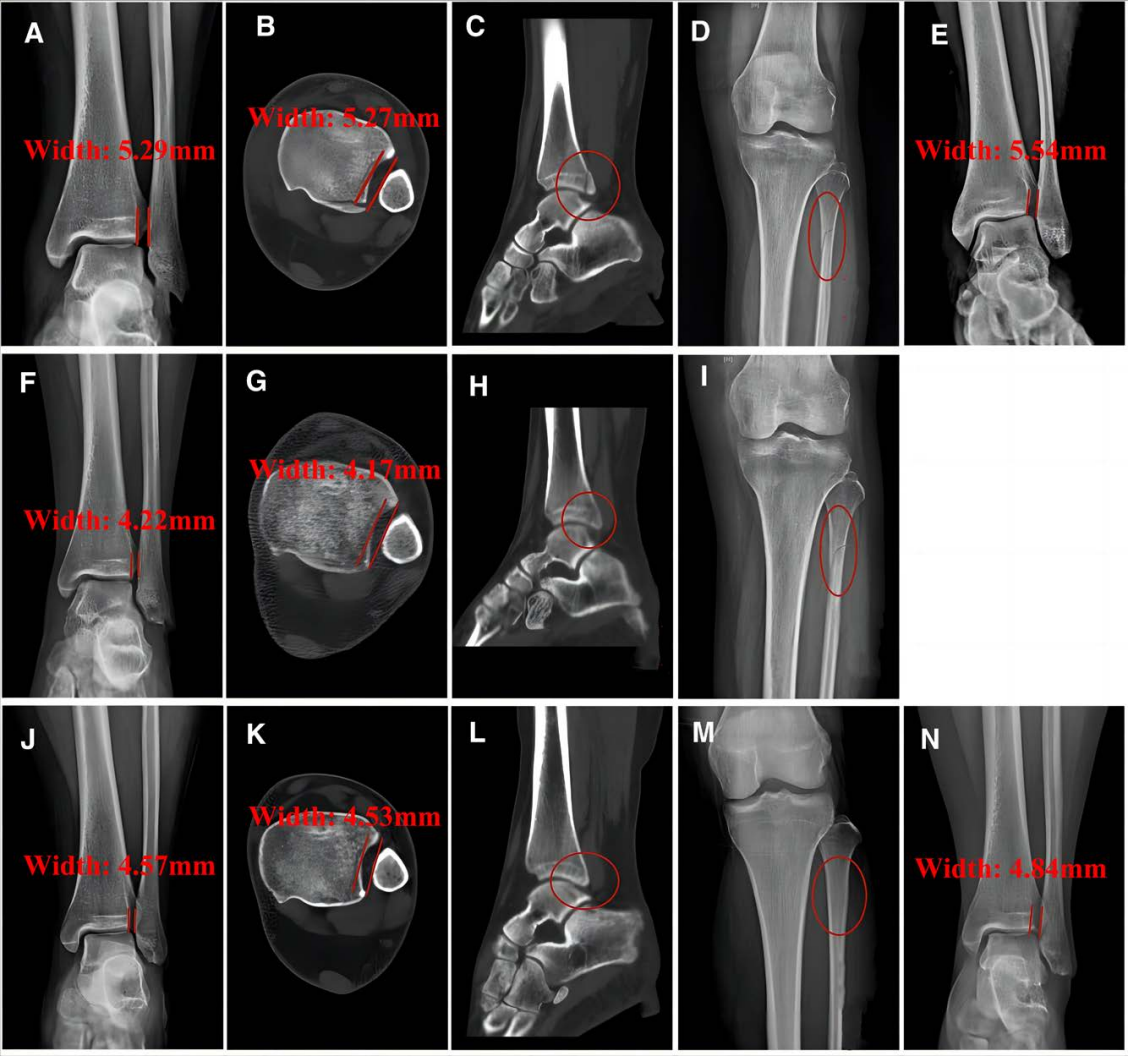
Typical imaging of the patient. (A–E) Radiography and CT scans at the first visit: (A) and (E) represented the non-stress and stress views, respectively; the width of the inferior tibiofibular space was 5.29 mm and 5.54 mm, respectively; (B) showed 5.27 mm in width of inferior tibiofibular space; (C) showed a fracture of the Volkmann tuberosity; (D) showed proximal tubular fracture. (F)–(I) Radiography and CT scans in 14th week after injury: (F) and (G) showed the width of the inferior tibiofibular space was 4.22 mm and 4.17 mm, respectively; (H) revealed the union of Volkmann fracture; (I) showed partial union of proximal tubular fracture, as the fracture line got obscure. (J)–(N) Radiography and CT scans at the 41st month follow-up after injury: (J) and (N) represented that the width of the inferior tibiofibular space on the non-stress and stress views was 4.57 mm and 4.84 mm, respectively; (K) showed 4.53 mm in width of inferior tibiofibular space in a cross section view of CT scans; (L) showed the total union of Volkmann fracture; (M) showed total union of proximal tubular fracture. CT = computed tomography.
